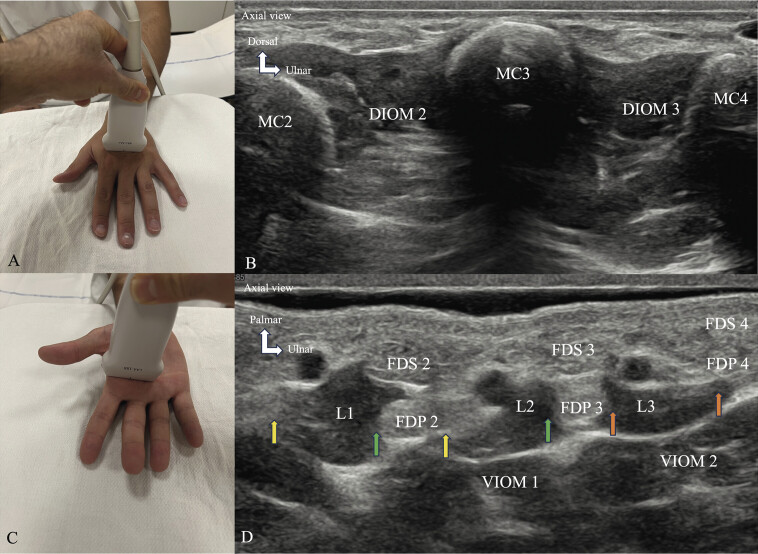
Normal ultrasound appearance of interosseous and lumbrical muscles. The probe is placed transversely at the level of the metacarpal shafts on the dorsal aspect of the hand to identify the DIOM (
